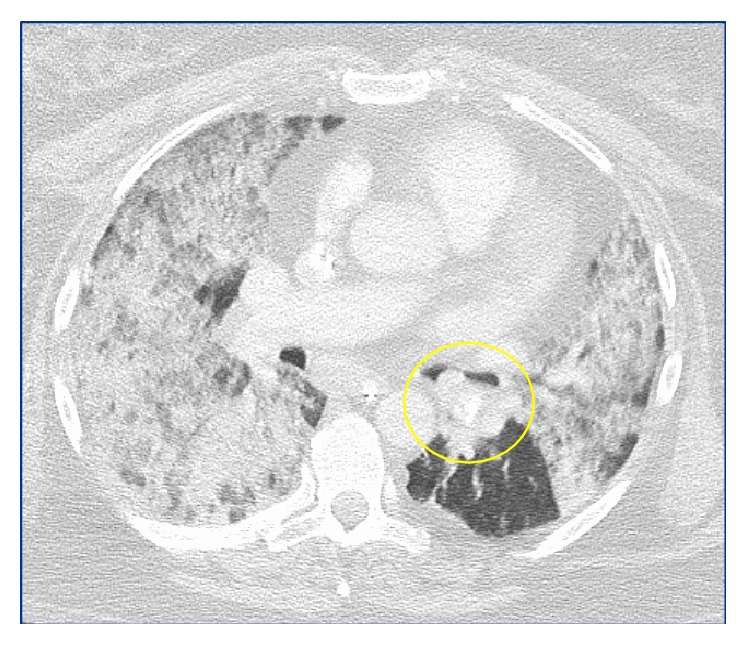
CTA chest showing bilateral ground glass opacities and highlighting the left endobronchial lesion (yellow circle).
